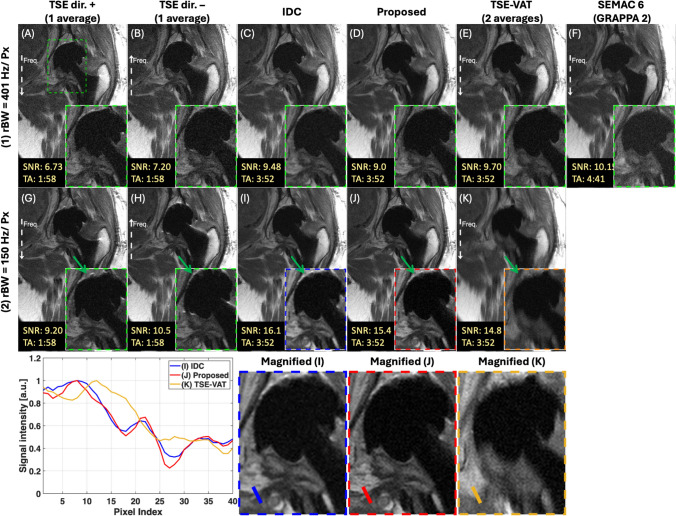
MR images of a 74-year-old female (BMI of 23.5 kg/m^2^) of left THA (titanium). aSNR and acquisition times (TA) are indicated in yellow in the lower-left corner. Frequency-encoding direction is labeled by white arrows. Opposite distortions are observed in the TSE acquisition pairs in (A)(B) and (G)(H). The zoomed-in images show that IDC, the proposed method, TSE-VAT and SEMAC correct in-plane distortion near the acetabular cup (green arrows). E, F, K Blurring is evident in TSE-VAT and SEMAC at both high and low bandwidths. Line profiles for the 120 Hz/Px case are drawn on small structures to compare signal transitions and smoothness among IDC, the proposed method, and TSE-VAT
